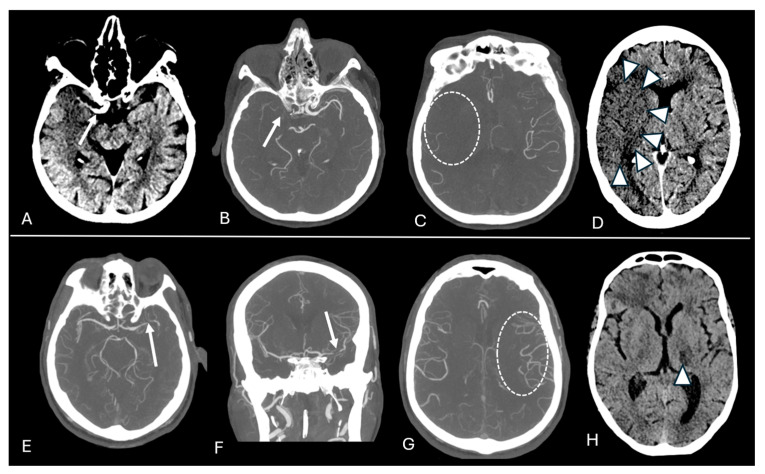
(A–D) A patient with a higher thrombus burden. (A) Axial NCCT and (B,C) CTA views show an occlusion in the right intracranial ICA segment (arrows). A poor collateral score is depicted (dashed circle). (D) An NCCT at 2-day follow-up shows a large final infarct volume in the right MCA territory (arrowheads). (E–H) A patient with a lower thrombus burden. (E–G) CTA views show a thrombus in the left distal M1 segment (arrows) and a good collateral score (dashed circle). (H) An NCCT at 1-day follow-up shows a small core volume (arrowhead) in the left thalamus.
